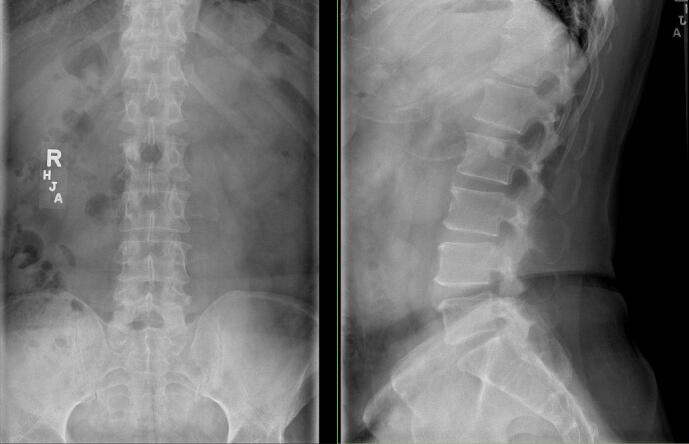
Anterior-posterior (AP) and lateral lumbar radiographs showing multilevel disc degeneration and lower lumbar spondylosis.
